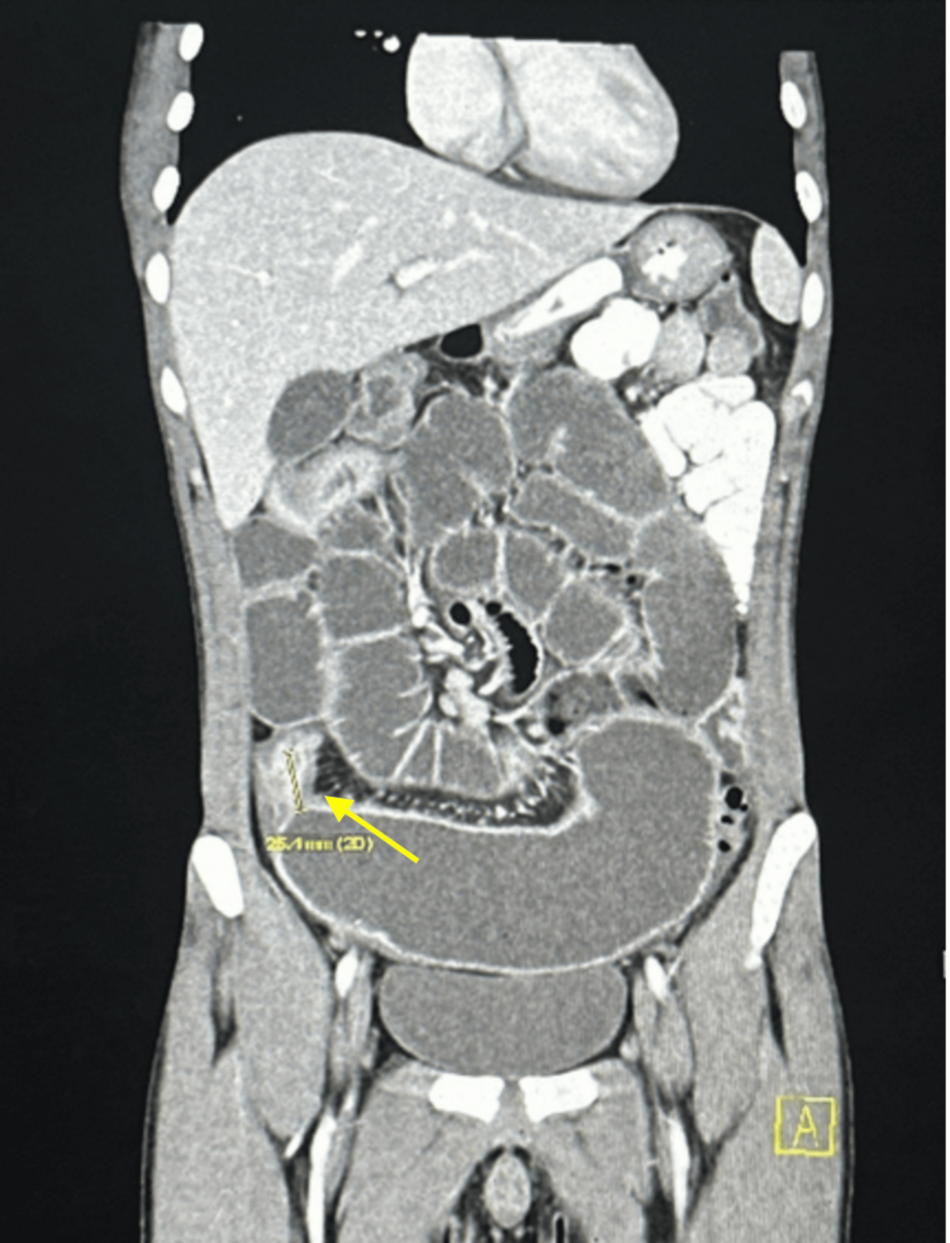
CT abdomen and pelvis showing active inflammatory small bowel Crohn’s disease with luminal narrowing measuring 2.5 cm in length and involving the distal and terminal ileum, with small bowel obstruction
